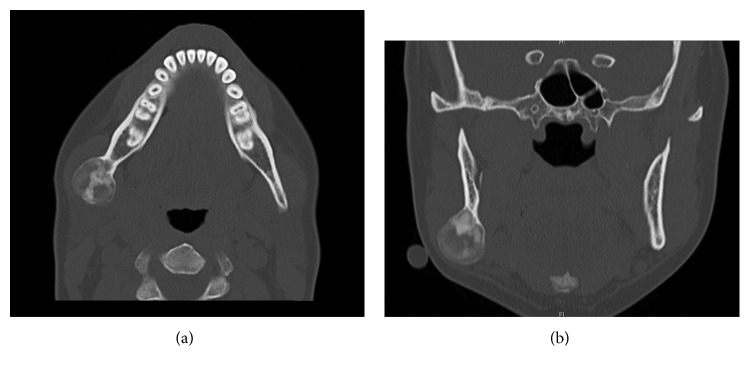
(a) CT axial cross section and (b) CT coronal cross section of an expansile mixed lesion of the right angle region of the mandible.
